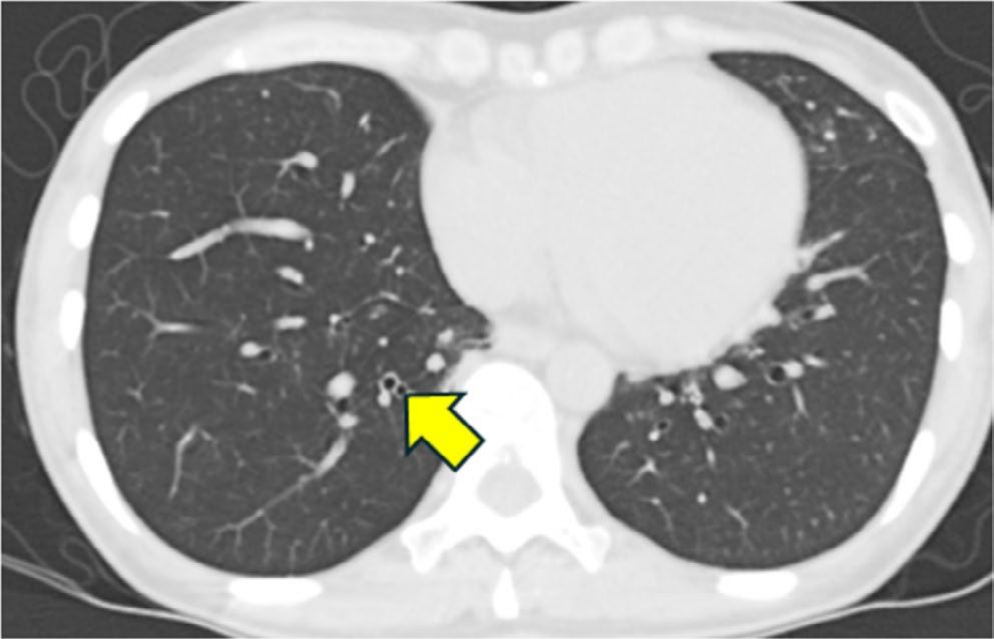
CT image taken on September 29, 2017, prior to administration of mepolizumab, showing bronchial wall thickening in the lower airway.
